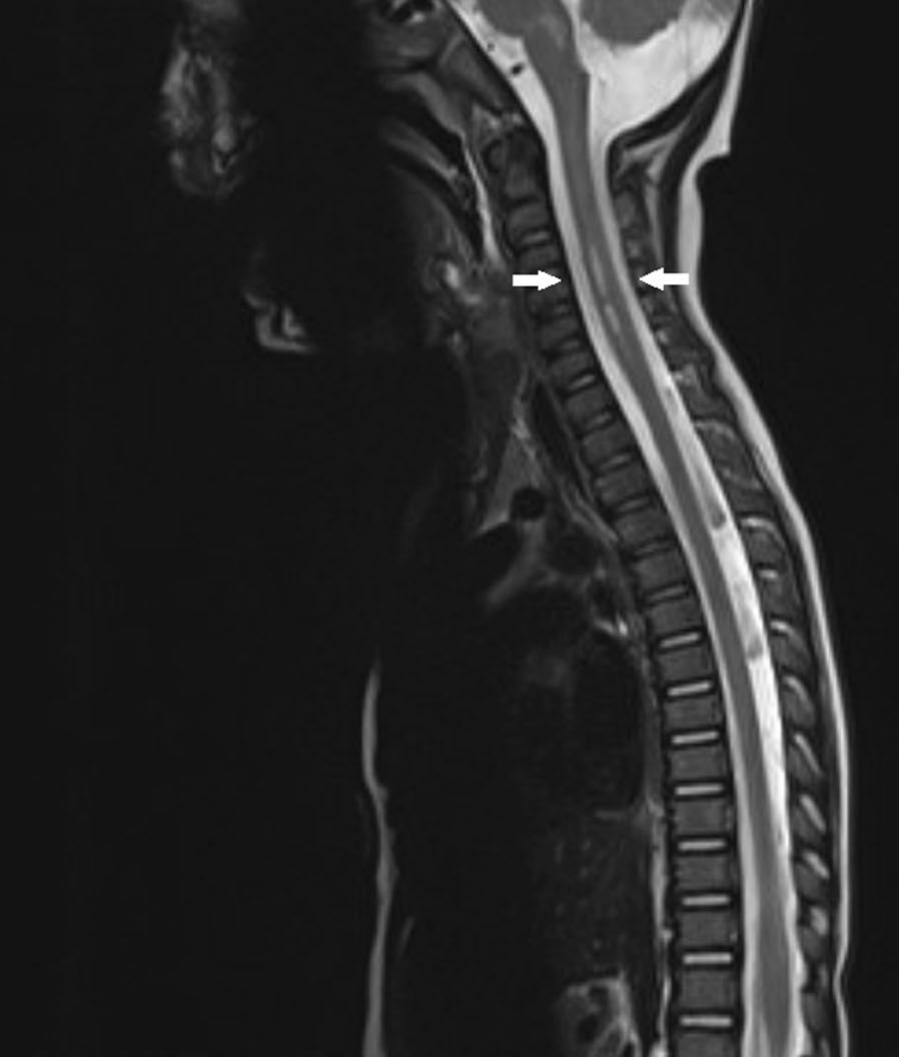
Sagittal T2-weighted magnetic resonance imaging of the spinal cord 1 week after onset of neurological symptoms demonstrated a longitudinal spinal cord lesion of the gray matter at the cervical level C3–C5. See arrows. These findings were consistent with rhombencephalomyelitis with radiculitis
